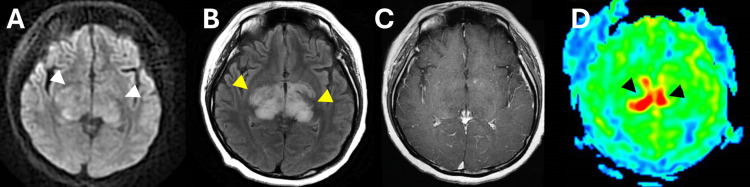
Magnetic resonance imaging (MRI) findings four months after the initial MRI. A) A diffusion-weighted imaging sequence revealed enlarged high-signal intensity areas (white arrowheads) in bilateral basal ganglia. B) A fluid-attenuated inversion recovery (FLAIR) image revealed enlarged high-signal intensity areas (yellow arrowheads) in bilateral basal ganglia. C) No contrast enhancement was observed on a gadolinium T1-weighted image. D) The arterial spin-labeling MRI revealed increased cerebral blood flow in narrower bilateral basal ganglia areas (black arrowheads) compared to the FLAIR image.
